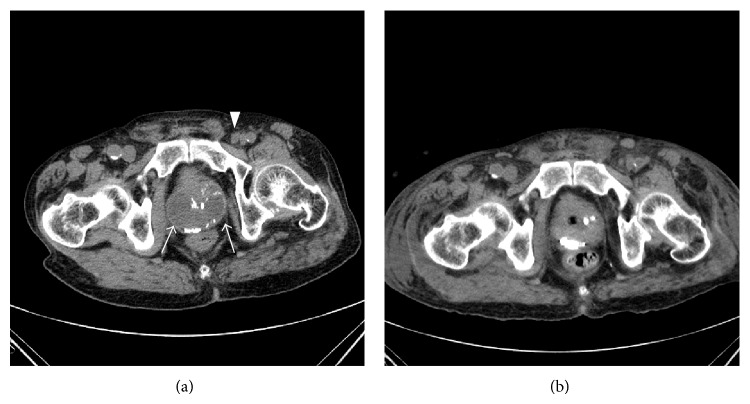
(a) CT revealed low-attenuating, round, well-demarcated fluid collection with calcification at the dorsal site of the prostate (arrows) and some lymph nodes were swollen near the prostate (arrowhead). The cystic lesion volume was about 50 mL. (b) CT revealed clear reduction of fluid collection at the dorsal site of the prostate.
